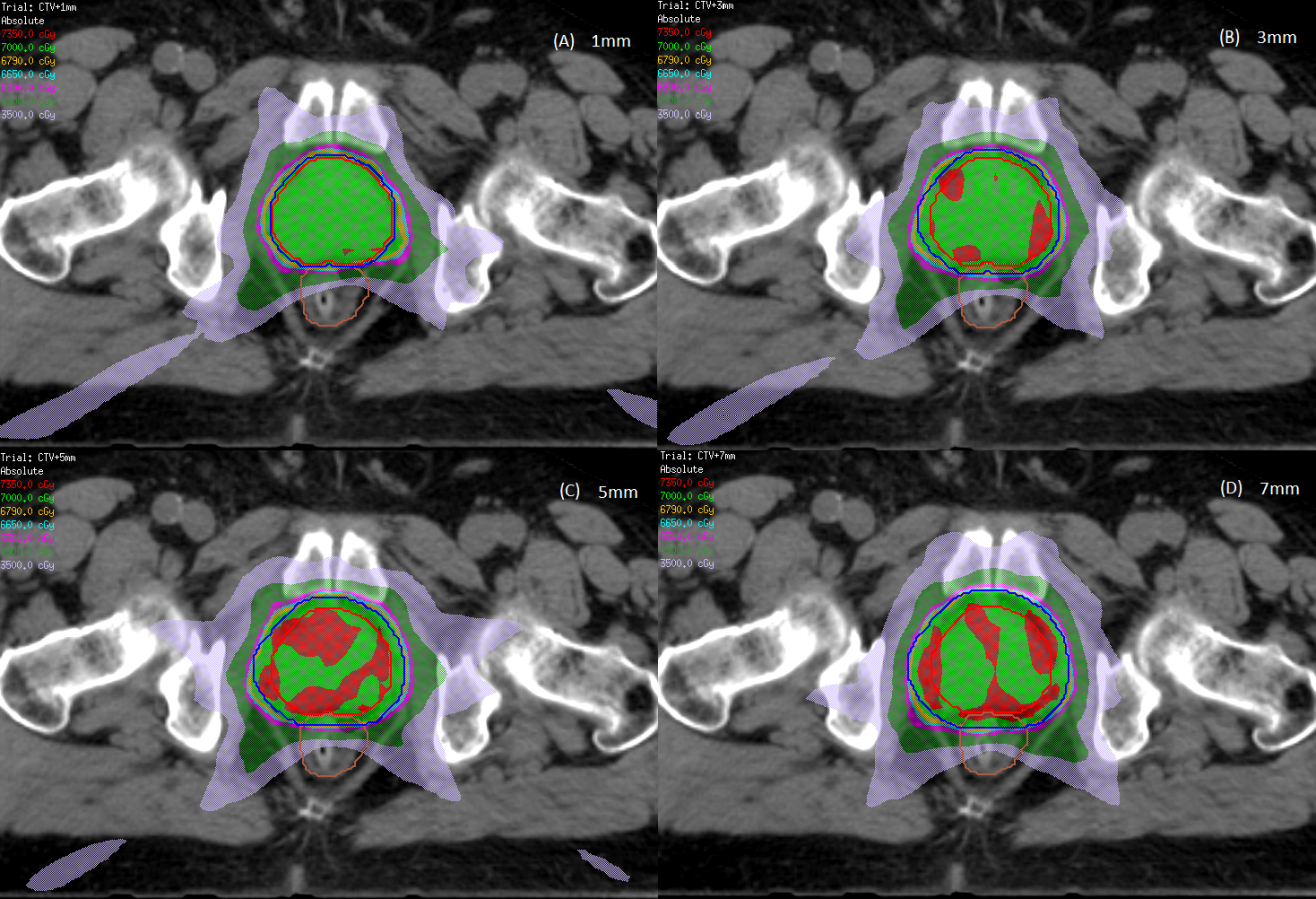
Comparison of the IMRT plan for 1, 3, 5, and 7 mm PTV margins on P‐CT.
